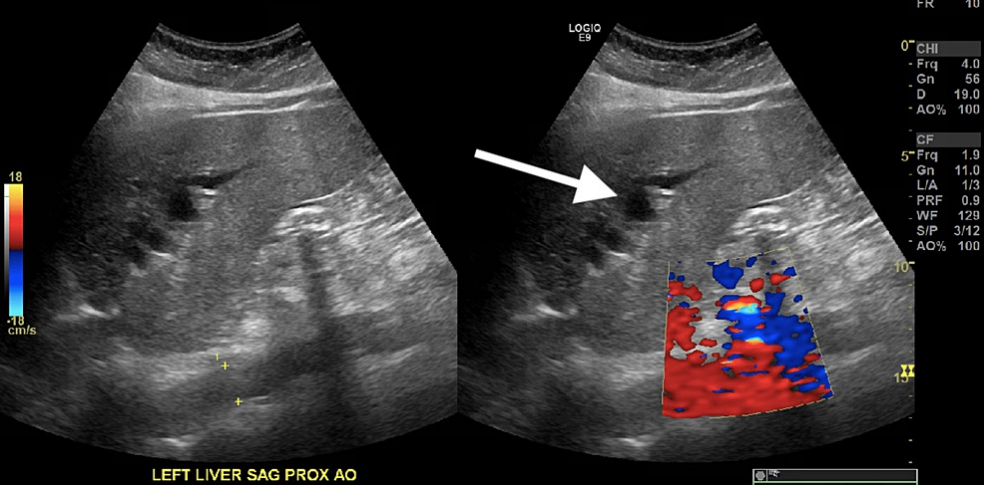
Ultrasound of the right-upper quadrant showing dilation of the bile ducts (arrow), which appear black in contrast to the blood vessels, which have a doppler signal
